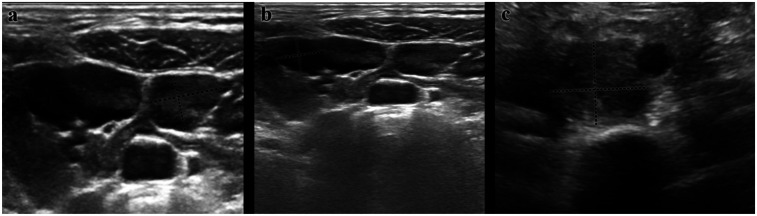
Representative ultrasonographic images of cervical lymph nodes. (a) Reactive LAP: Oval shape, preserved echogenic hilum, thin cortex, and regular margins; Doppler shows hilar vascularity. (b) Suspicious LAP: Rounded morphology, cortical thickness >3 mm, heterogeneous echotexture, and mixed hilar/peripheral vascularity. (c) High suspicion of malignancy LAP: Loss of hilum, irregular margins, marked cortical thickening, and predominant peripheral vascularity.
